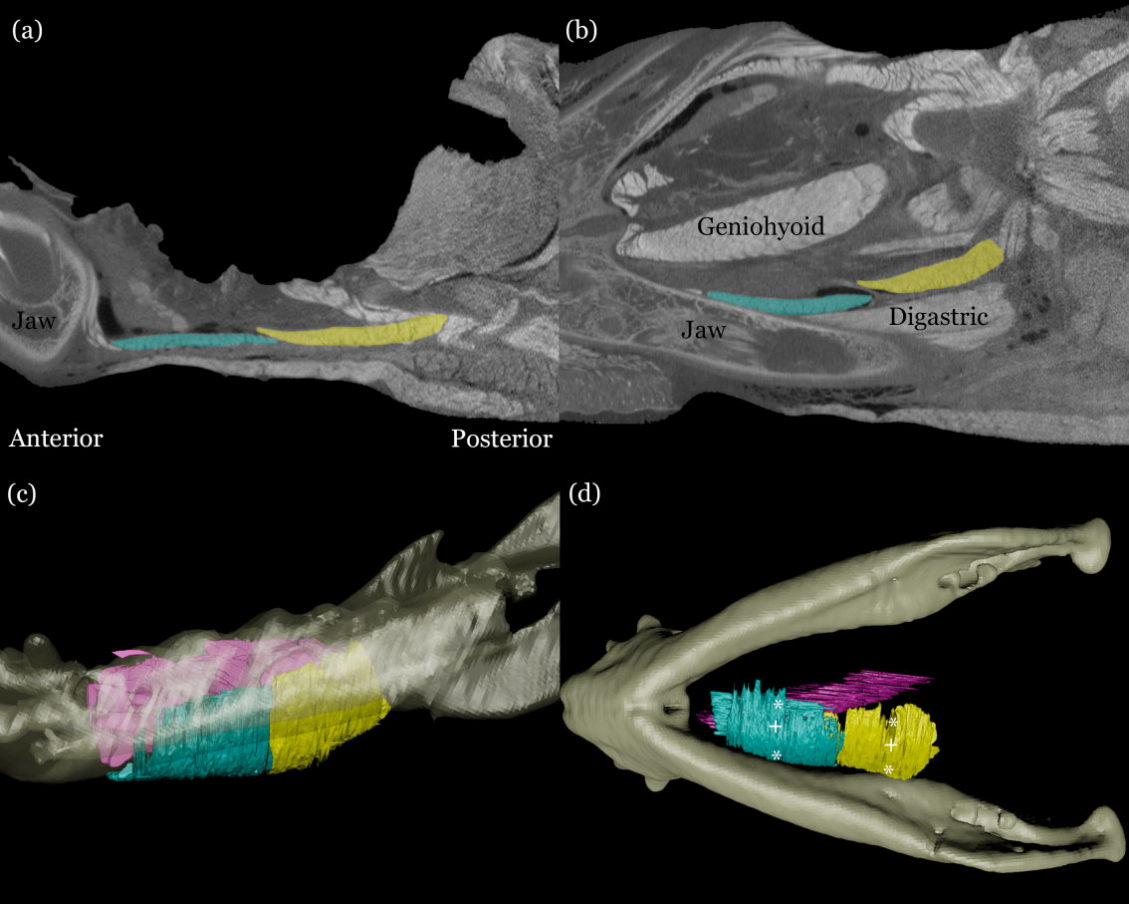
Contrast-enhanced CT scan with an anteroposterior slice (a, left is anterior), dorsoventral slice (b, bottom is ventral), and lateral (c, left is anterior) and ventral (d) three dimensional renders of anterior mylohyoid (blue), posterior mylohyoid (Yellow) and genioglossus (pink). The symbol * in D represents locations of bead placement for fluoromicrometry; + and ^ in D represent placement of electrodes for EMG in mylohyoid and genioglossus respectively. Labels in (a) and (b) are provided for reference.
